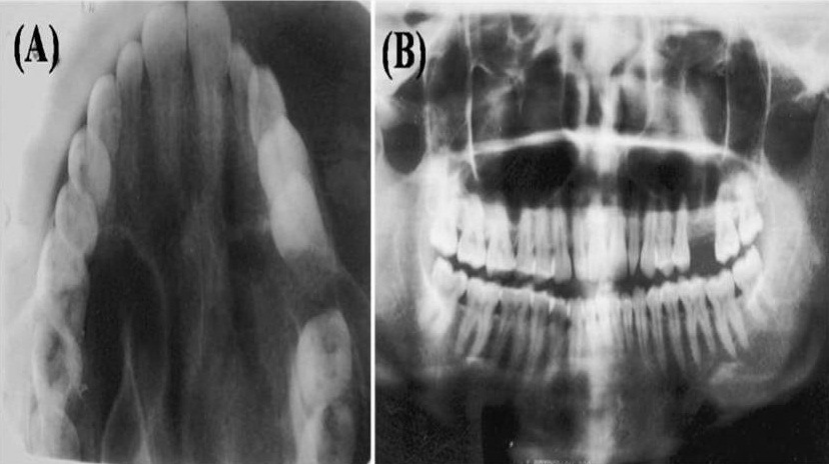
(A) maxillary occlusal radiograph; (B) panoramic radiograph
